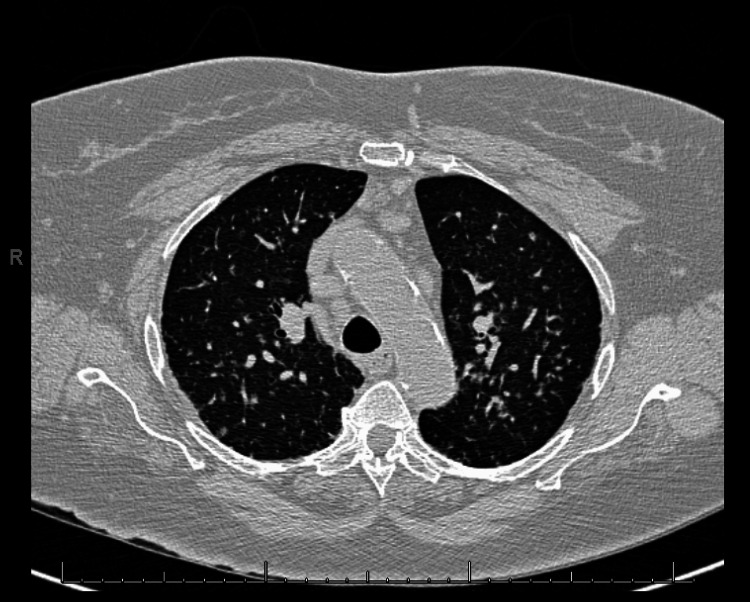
High-resolution CT imaging of the chest revealing numerous bilateral pulmonary nodules accompanied by extensive mediastinal and hilar lymphadenopathy
